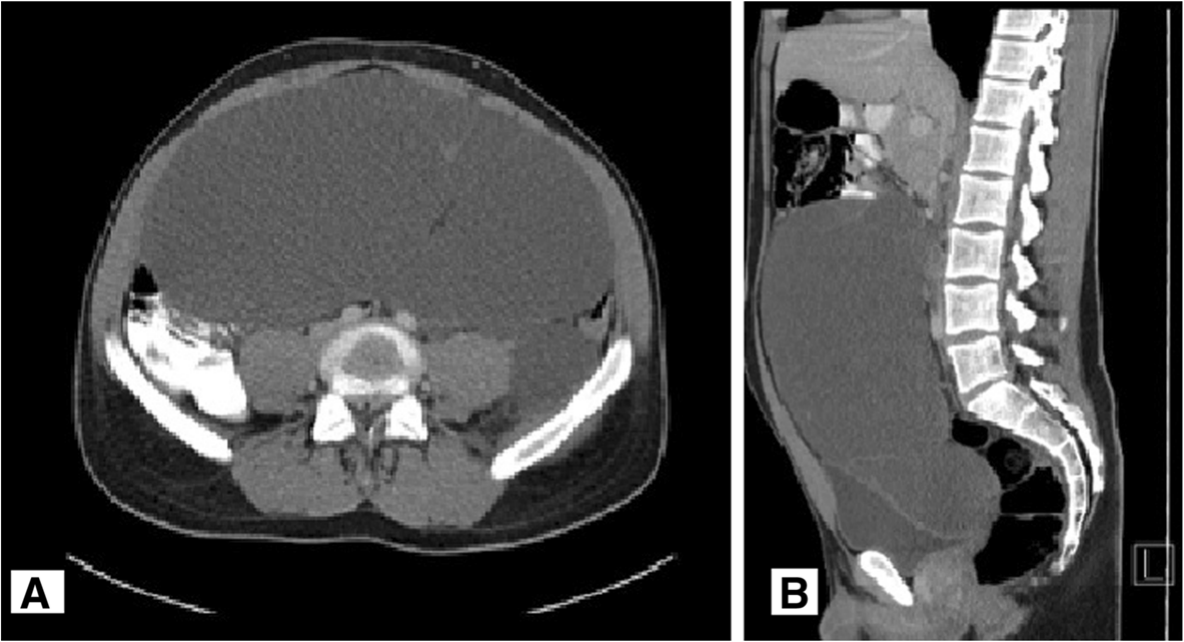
Oral and intravenous contrast-enhanced computed tomography (CT) scans. (A) Transverse and (B) sagittal imagesshowing a large abdominal tumorous mass.
